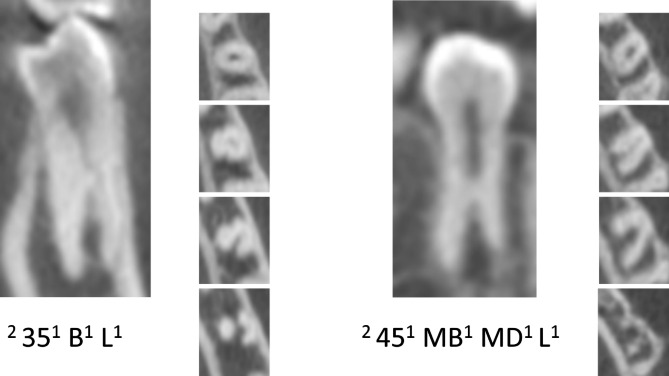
CBCT View (Sagittal and axial) of mandibular second premolars showing the root and canal variations
